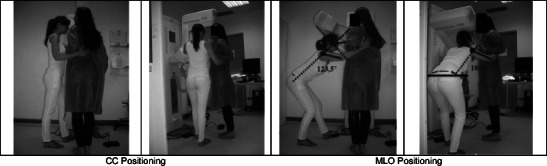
Postures assumed by the radiographer with similar height compared to the patient
